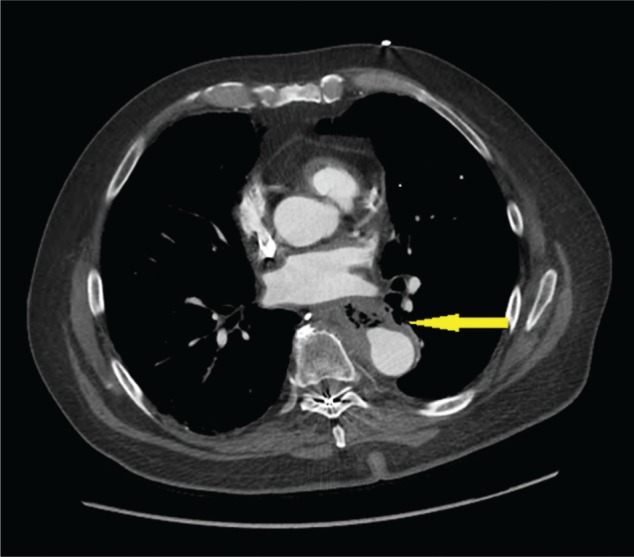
CT angiography of the chest. The arrow shows out-pouching of the intravenous contrast within the descending thoracic aorta, with marked mural thickening of the adjacent esophagus, with extra luminal foci of air between the esophagus and the aorta.
